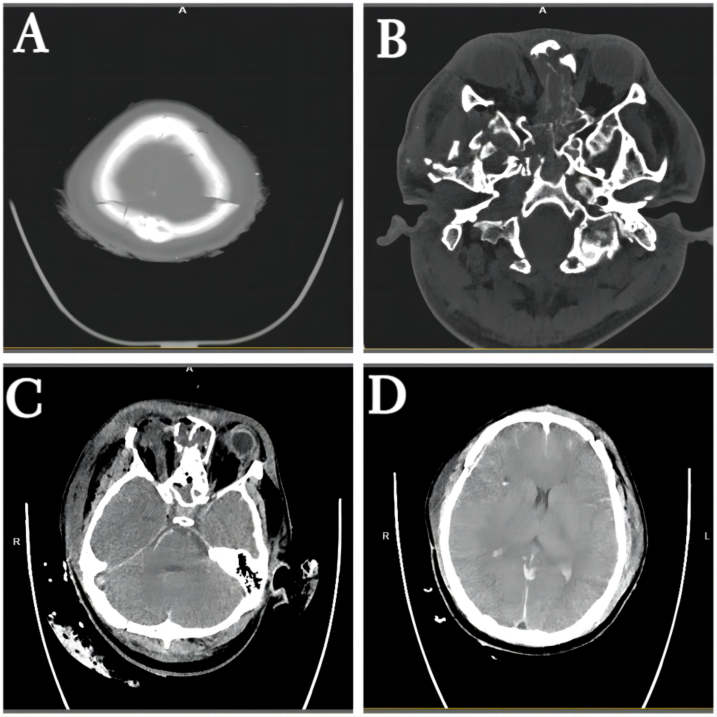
(A and B and C and D)The first head CT image upon the patient's admission.
